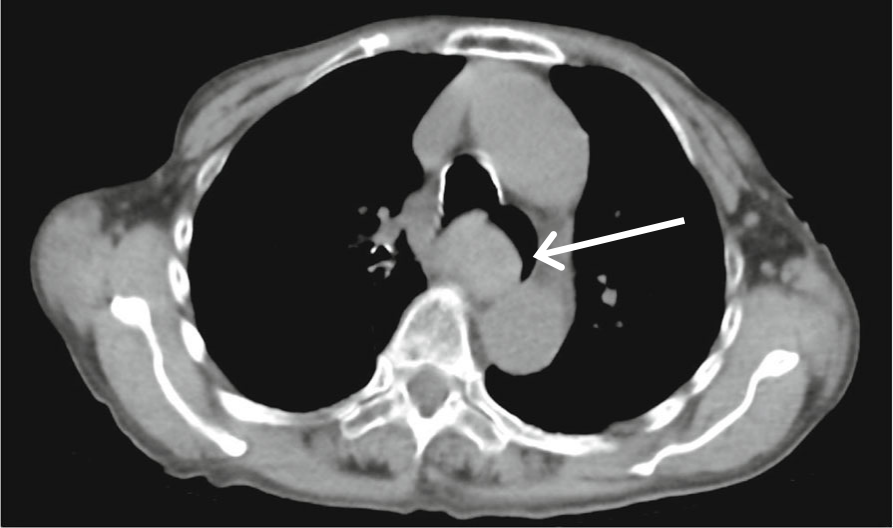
Computed tomography thoracic examination immediately after hematemesis. The thoracic oesophagus is dilated. The white arrow indicates the oesophagus
